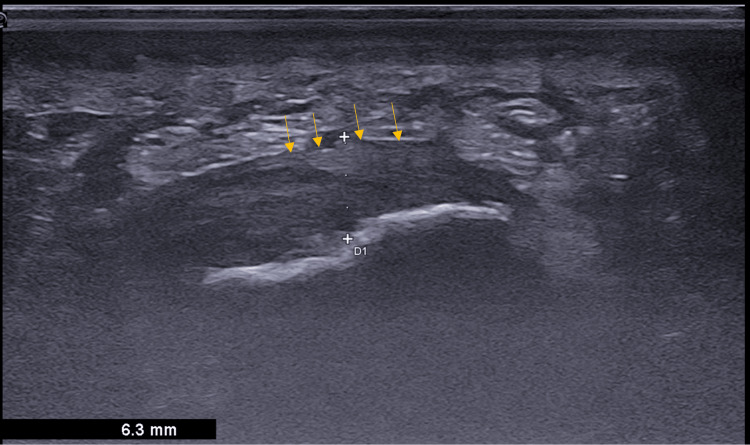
Sagittal B-mode ultrasound image of a 38-year-old female patient with plantar fasciitis shows hypoechoic, thickened plantar fascia measuring 6.3 mm (arrow showing the region of interest) with loss of the fibrillar pattern.
